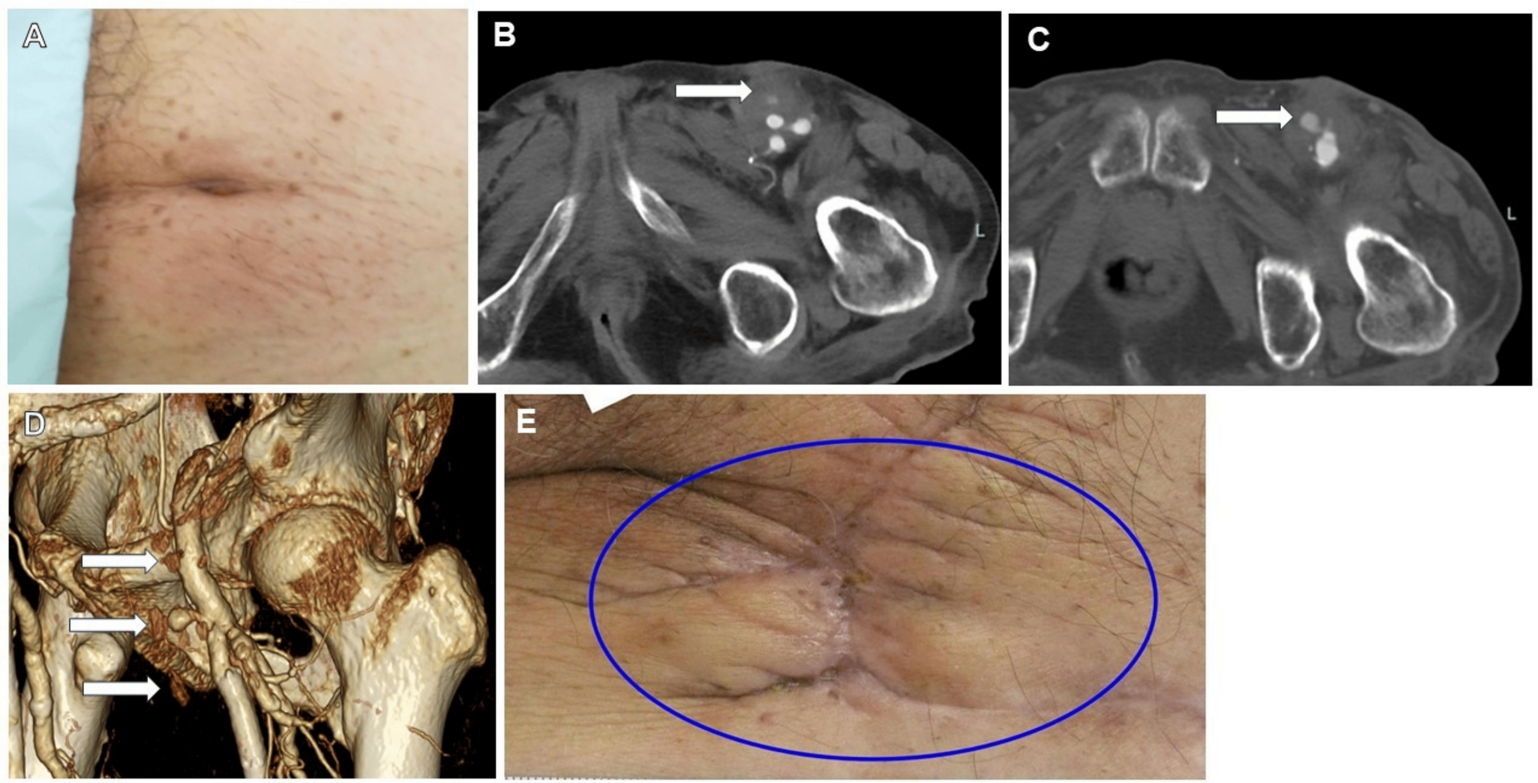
Puncture site photographs and CT images of case 1. A: Photograph of the puncture site at the left femoral artery (FA) after removal of the suture. There was only mild redness and swelling, and blood tests showed no signs of inflammation. B: Contrast CT scan taken when the patient presented to the hospital with bleeding from the puncture site. A pseudoaneurysm (arrow) was found at the anterior aspect of the FA, and a soft tissue shadow with low contrast was observed around it. C: CT image of the second infected pseudoaneurysm. A larger aneurysm than the first was observed (arrow), and a low-contrast soft tissue shadow was seen around it. D: 3D CT image of the second infected pseudoaneurysm. An oozing hemorrhage was also seen around the pseudoaneurysm (arrows), suggesting an extension of the area of infected tissue. E: Photograph of the wound after the second vascular repair and skin flap surgery. There is scarring but no evidence of infection (circle). CT, computed tomography
